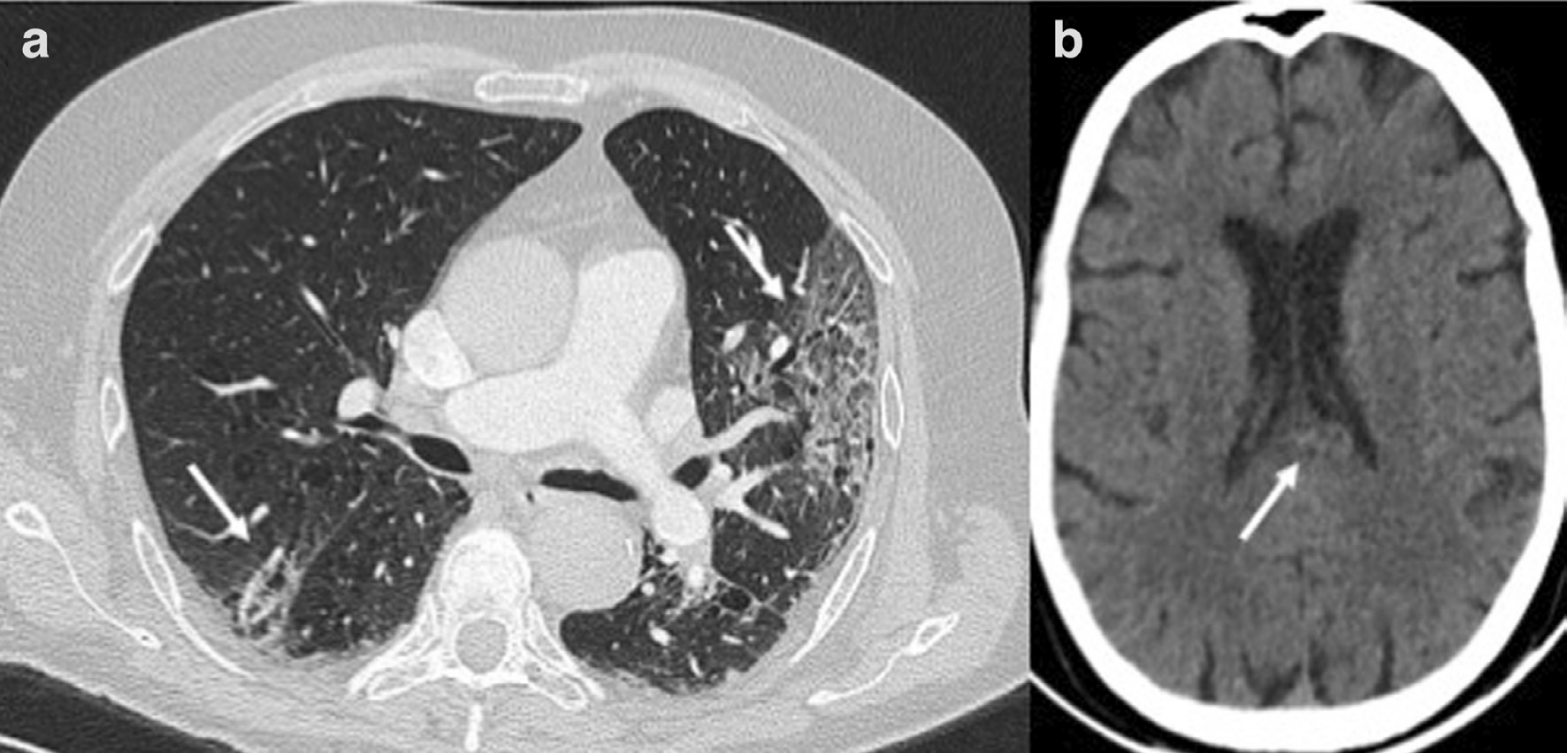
CT findings:( A) Chest CT shows bilateral ground glass opacities with a typical “crazy paving” pattern. (B) Brain CT shows a slightly hyperdense irregular lesion with slight mass effect on the medial wall of the occipital horn on the left lateral ventricle.
